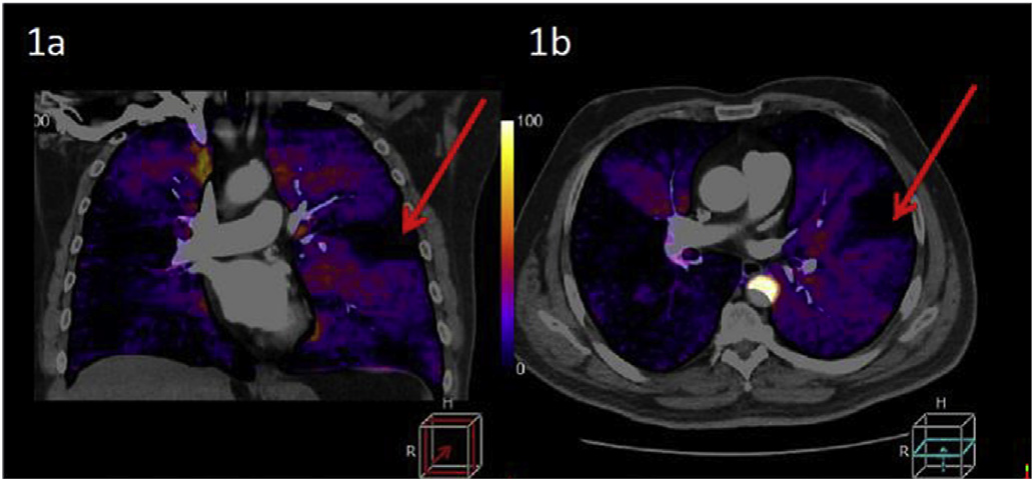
Coronal (1a) and axial (1b) CT-LSIM showing a classical triangular segmental perfusion defect (red arrows). (For interpretation of the references to colour in this figure legend, the reader is referred to the Web version of this article.)
